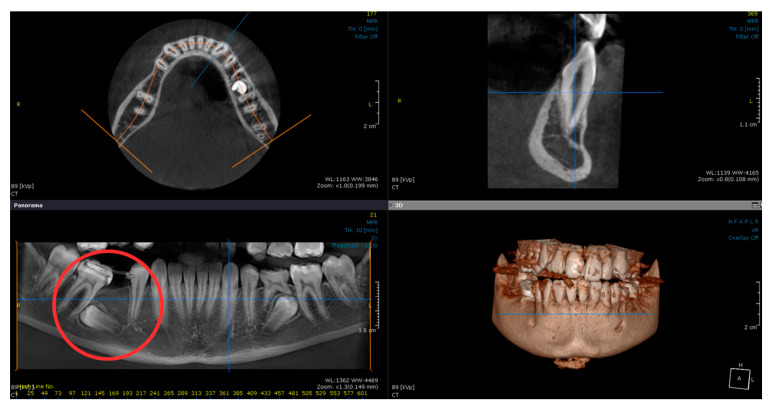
Mandibular CBCT showing the distal axial inclination of teeth 45 and 35.
